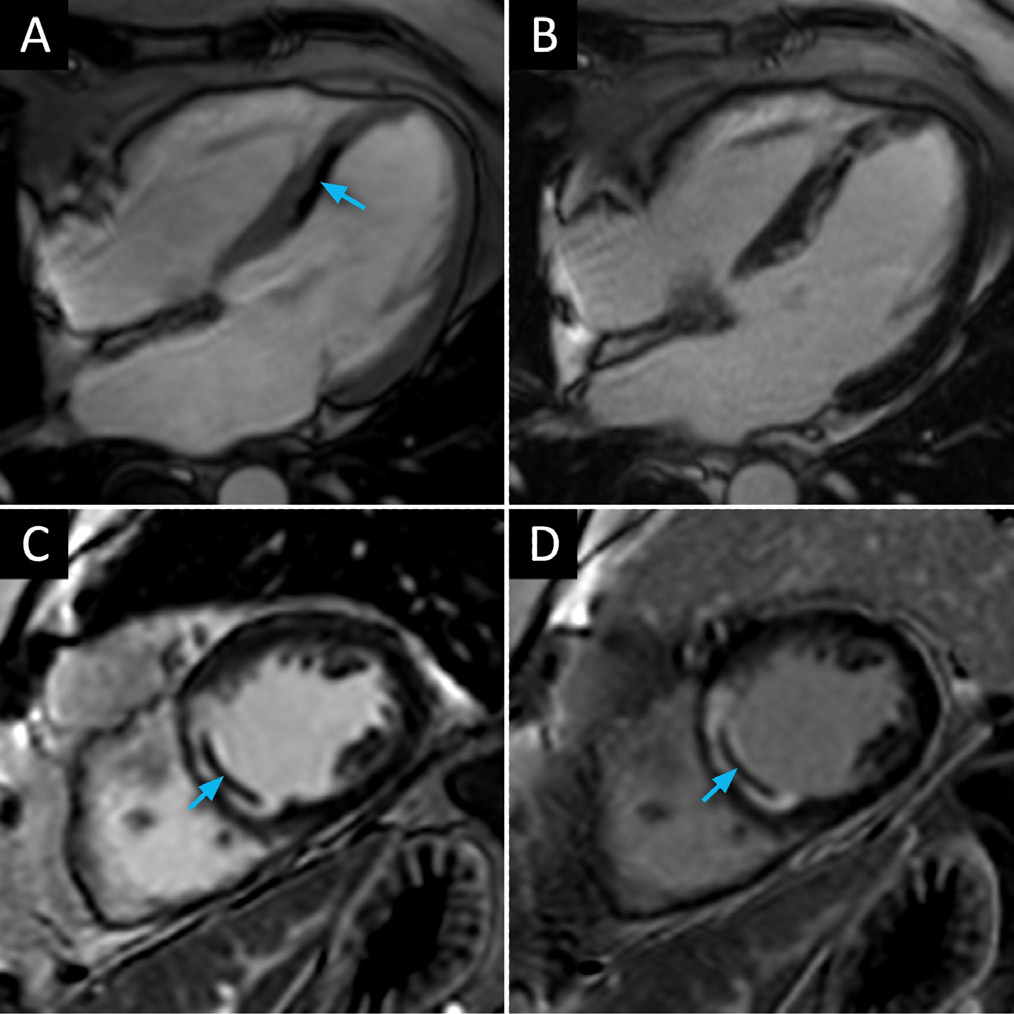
Contrast-enhanced cardiac MRI of a microvascular obstruction-like lesion (A) EGE image acquired in four-chamber view. (B) Conventional bright-blood LGE image acquired in four-chamber view. (C) Conventional bright-blood LGE image acquired in short-axis view. (D) Novel dark-blood LGE image acquired in short-axis view. The septal hypoenhancement is indicated by blue arrows. While there was no clear delineation of scar on bright-blood LGE in panel C, mimicking wall thinning and adjacent thrombus, there was an apparent ischemic scar with ‘dark core’ seen on dark-blood LGE, in keeping with a microvascular obstruction-like lesion. EGE, early gadolinium enhancement; LGE, late gadolinium enhancement.
